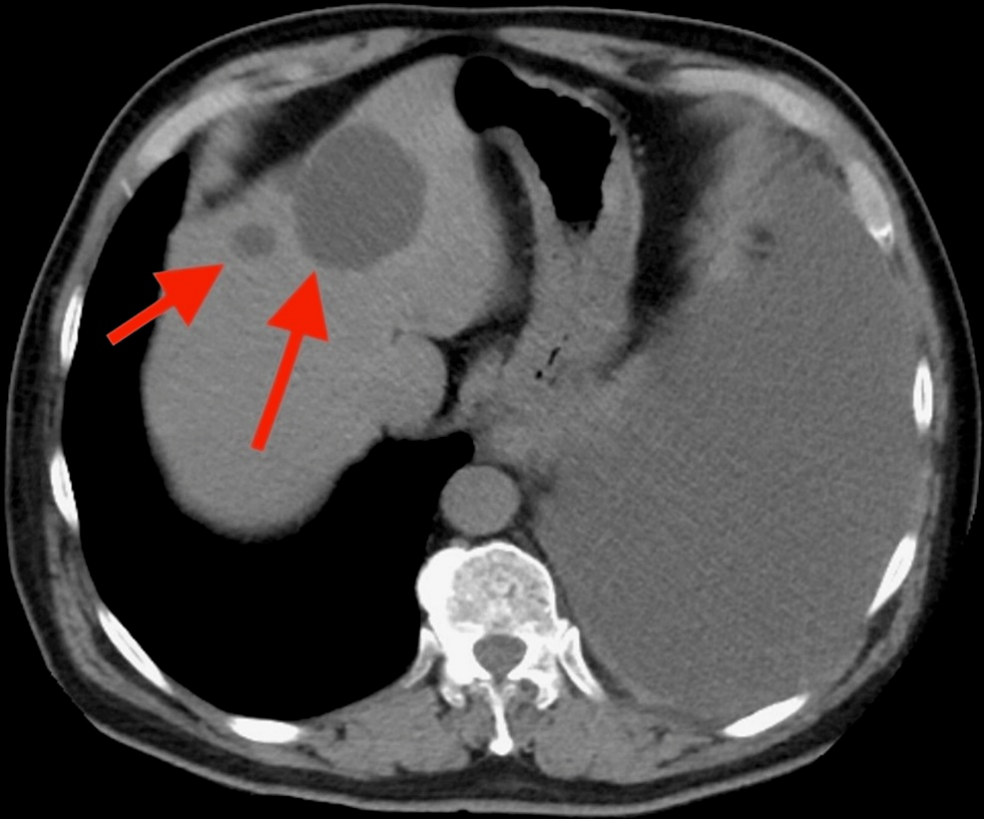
CT chest without contrast showing the upper part of the abdomen. CT without contrast shows multiple liver masses most likely neoplasms (red arrow).
